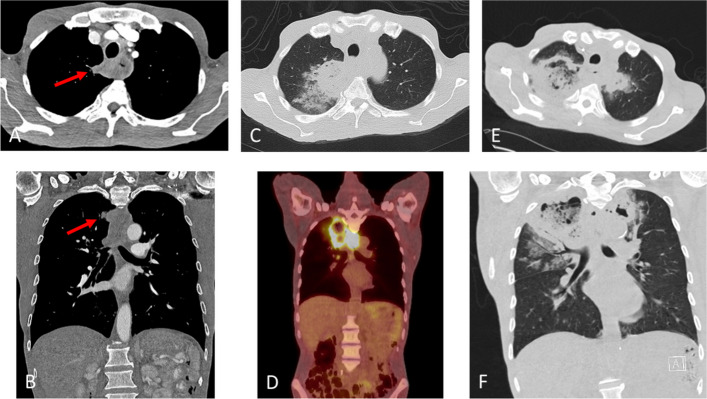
A, B Initial chest CT showing a soft tissue mass in the proximal esophagus with small nodular invasion to the right upper lobe. C, D PET/CT 3 weeks later showing a large necrotic mass in the right upper lobe concerning for an extending abscess from the adjacent esophageal lesion. E, F Chest CT at the emergency room 1 week after induction chemotherapy and 3 months after initial imaging showing an expanding right upper lobe cavitary mass
